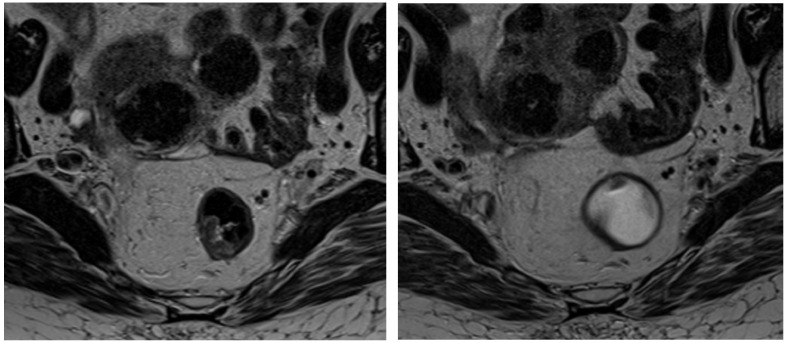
Staging MRI of rectal cancer before CRT (left image) and follow-up MRI after treatment (right image).
